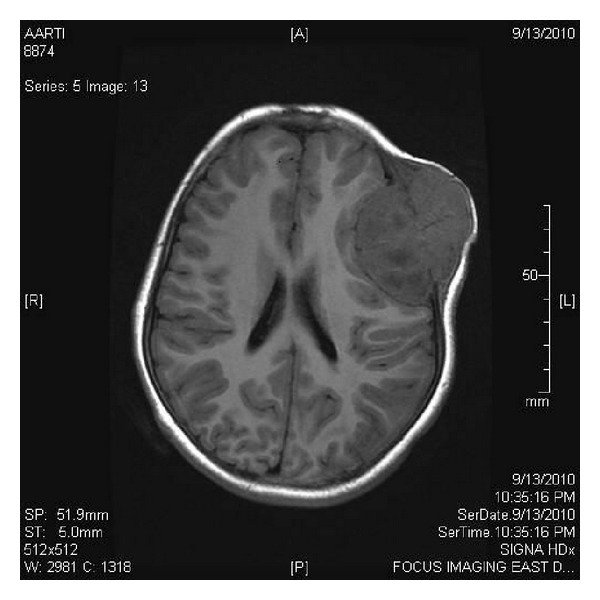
Postcontrast T1 weighted MRI showed intense, homogenously enhancing intracranial mass causing destruction of bone, with extradural soft tissue extension.
